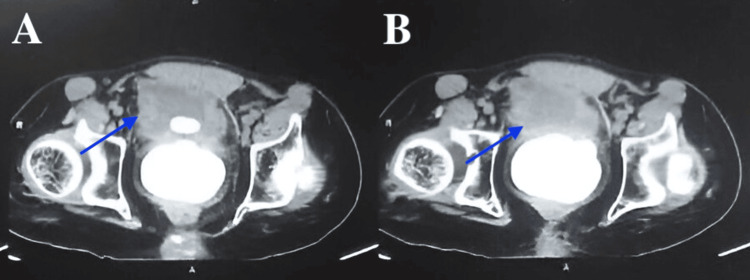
Non-contrast CT scan of the pelvis showing a massive prostate stone measuring approximately 4 cm within the prostatic urethra, causing significant obstruction (A, B). Blue arrows indicate the location of the stone within the urethra.
